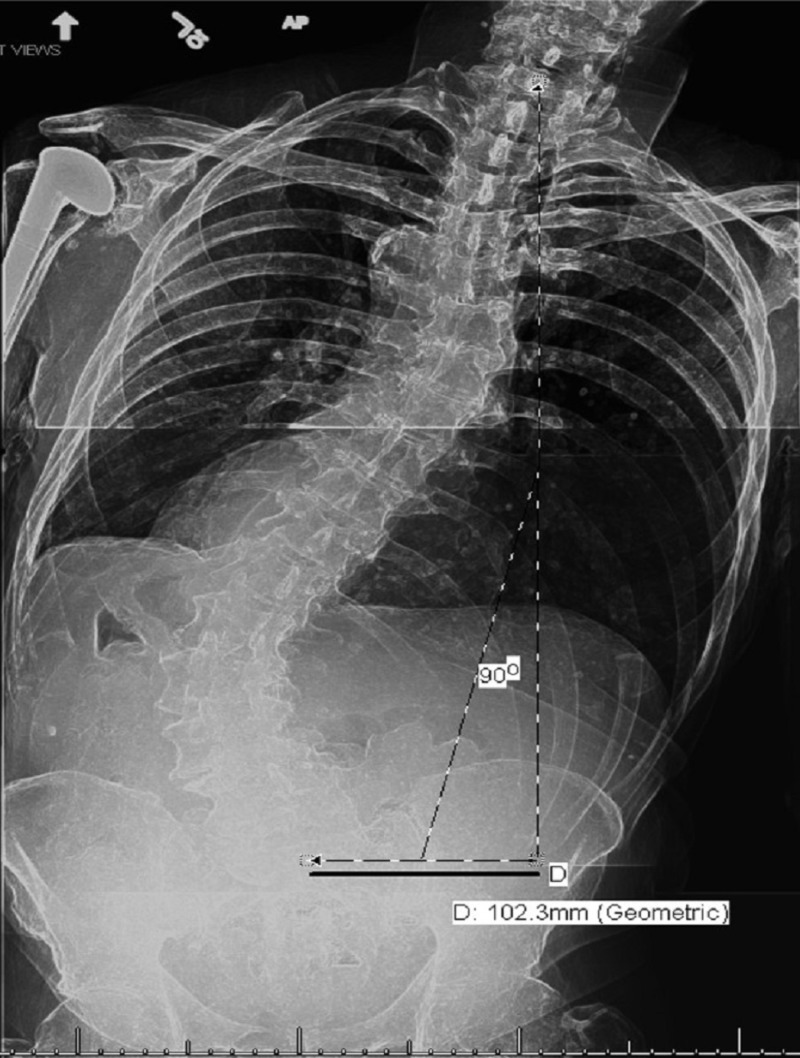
Erect scoliosis AP spine X-ray with coronal plumb line.
